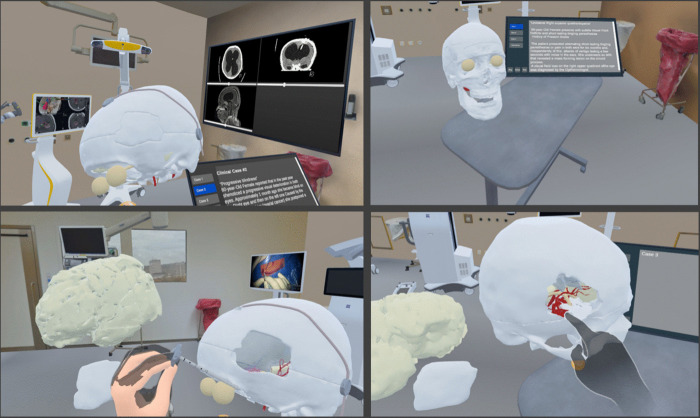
Interactive case-based learning environment displaying 3D anatomical models, case descriptions, an integrated MRI/CT viewer, and CAD models of medical implants for improved visualization and interaction
